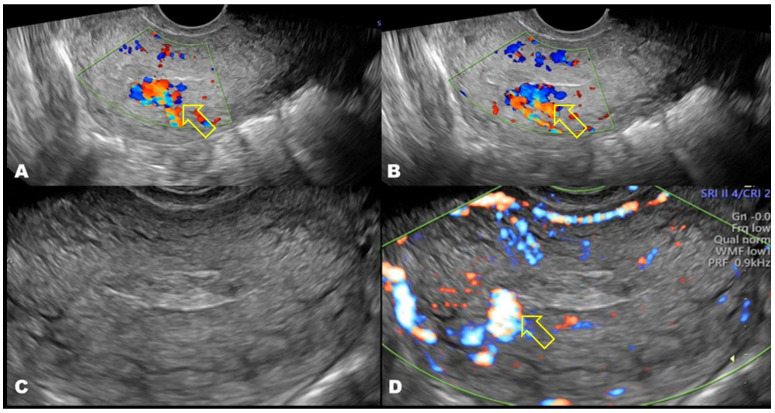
Repeat transvaginal ultrasound with color Doppler demonstrating acquired uterine arteriovenous fistula/malformation. (A,B) Longitudinal views showing intense myometrial hypervascularization with serpiginous vessels along the posterior uterine wall (indicated by a yellow arrow). (C,D) Transverse views demonstrating a mosaic pattern of turbulent, high-velocity, low-resistance flow consistent with arteriovenous shunting (indicated by a yellow arrow). AVF, arteriovenous fistula; AVM, arteriovenous malformation.
